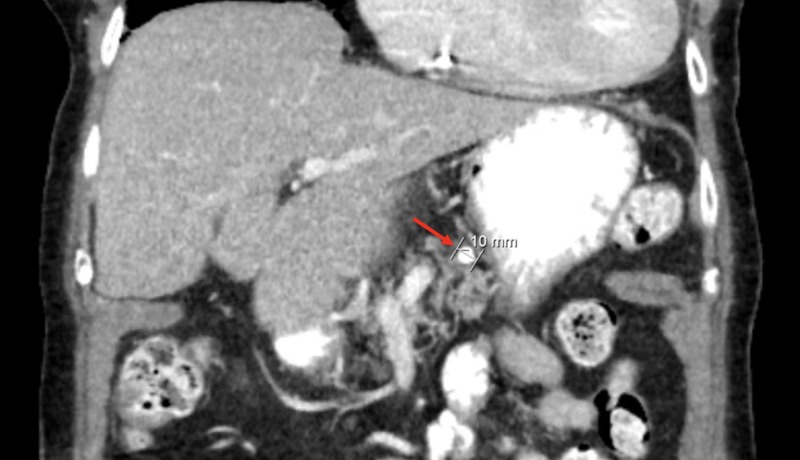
Computed tomography of the abdomen in coronal view showing a 10 mm pancreatic calculus (red arrow)
